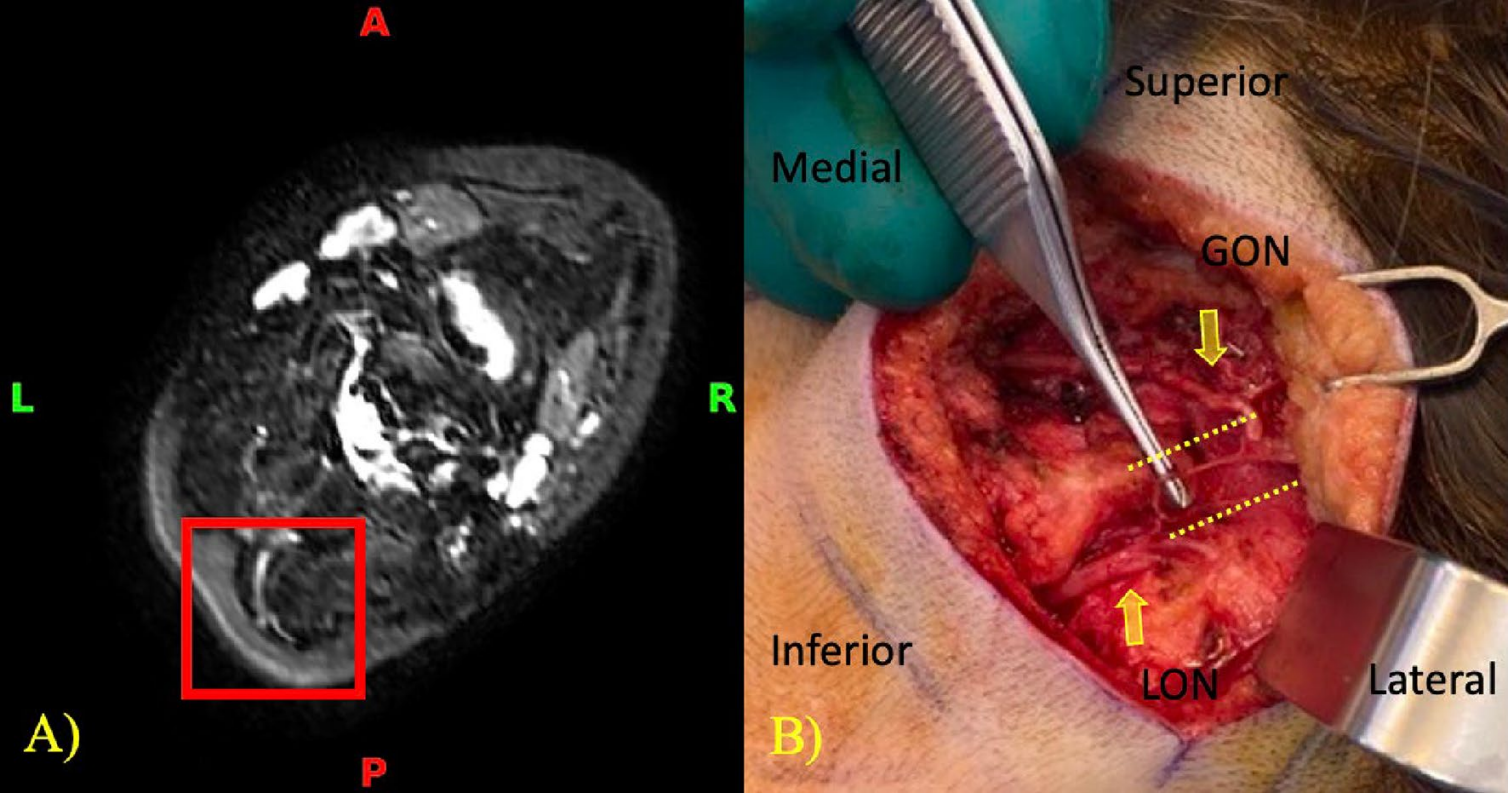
(A) Transverse NerveView MRI showing connected structures (red box), appearing to depict the right GON and LON. (B) Intraoperative image showing the GON and the LON (yellow arrows) having a neural connection (yellow dotted lines).
